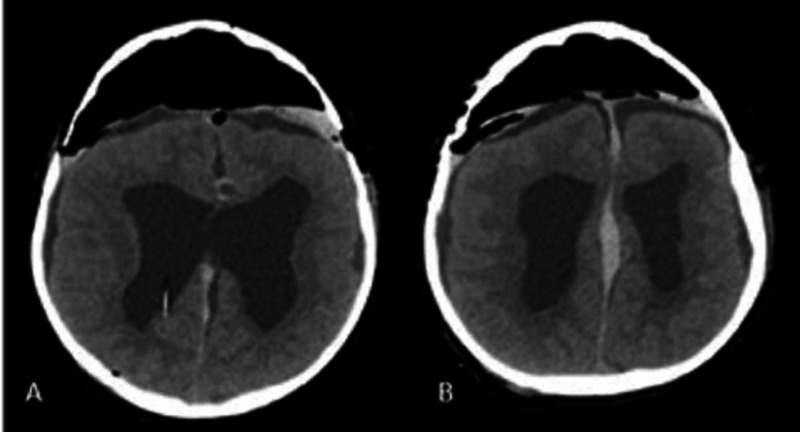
Post-operative non-contrast Brain CT scan, axial view A: Remaining frontal pneumocephalus. B: Interhemispheric hemorrhage and larger ventricles.
